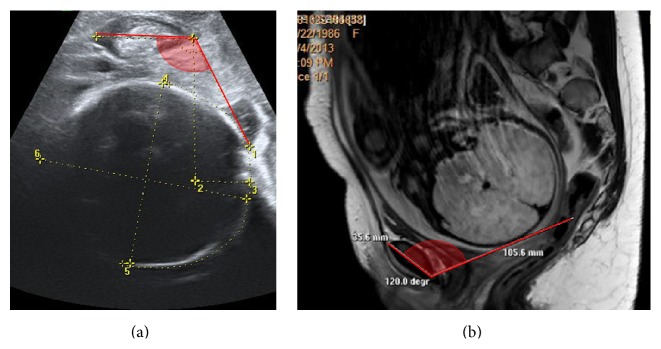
Measurement of the AOP in sagital TPU (a) and MRI (b) views, showing similar values, 120°.
